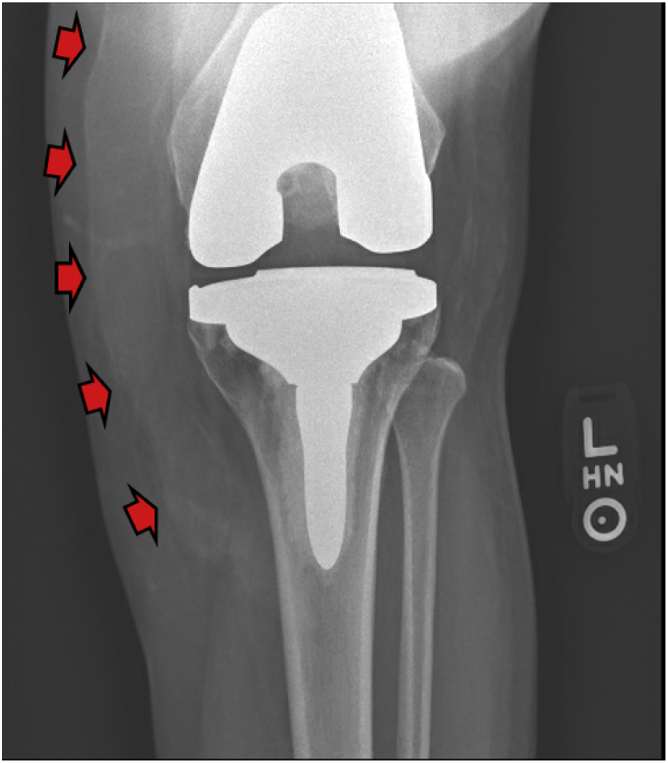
X-ray image showing swelling in the medial portion of the knee. Red arrows demonstrate effusion.
